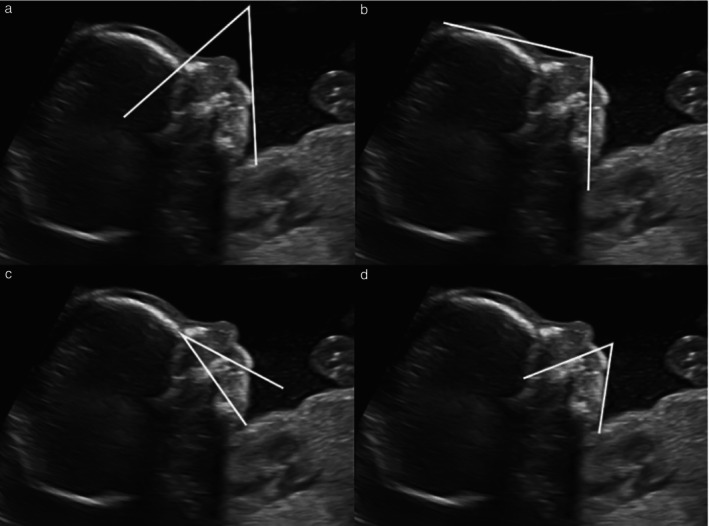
Two‐dimensional grayscale ultrasound images showing quantitative angle measurements on the facial profile of a fetus at 22 weeks' gestation diagnosed antenatally with suspected micrognathia. (a) Inferior facial angle: angle between line perpendicular to forehead and line joining mentum and protrusive lip. Diagnostic threshold for micrognathia, < 50°. (b) Fronto–naso–mental angle: angle between line from most prominent point of bony part of fetal forehead (os frontale) to tip of visible soft tissue of nose and line from most prominent point of soft tissue of fetal chin (mandible) to tip of visible soft tissue of nose. Diagnostic threshold for micrognathia, < 142°. (c) Maxilla–nasion–mandible angle: angle between intersection of maxilla–nasion and mandible–nasion lines. Diagnostic threshold for micrognathia, > 17°. (d) Facial maxillary angle: angle between line overlying maxilla and line across mentum tip and upper lip. Diagnostic threshold for micrognathia, < 66°.
